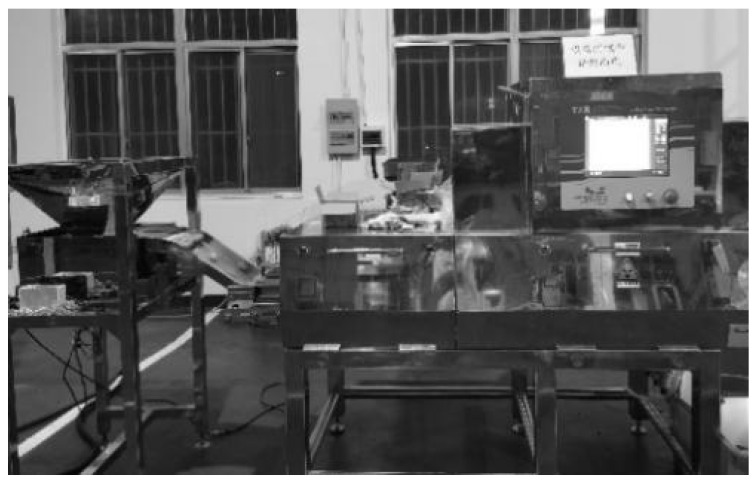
The X-ray imaging and walnut grading system.
